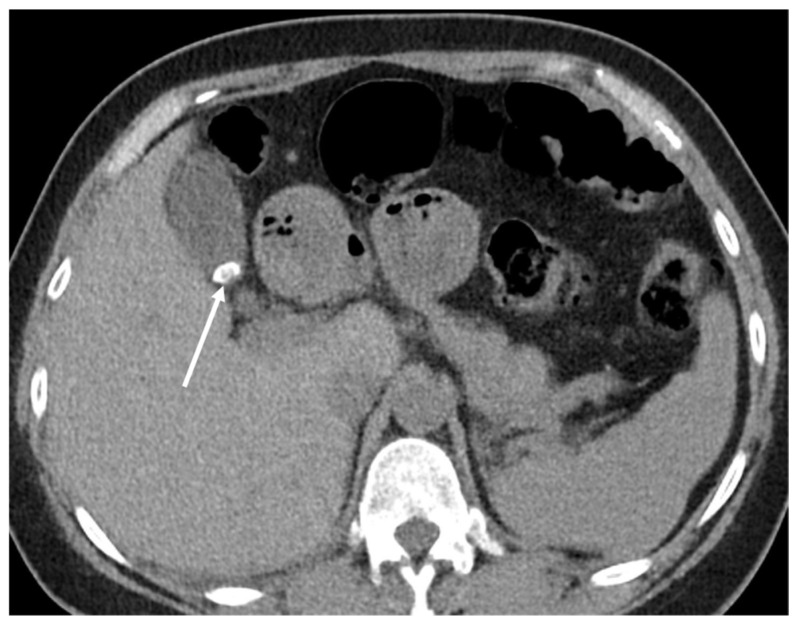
An axial low-dose computed tomography scan of a 52-year-old female demonstrating an incidental gallstone (arrow).
